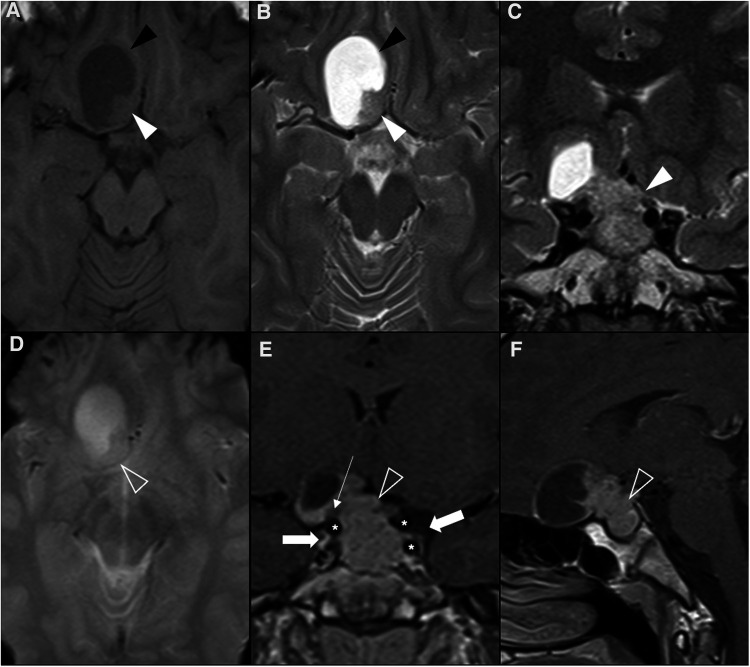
Prolactin-releasing pituitary neuroendocrine tumor in a 16-year-old boy presenting with headaches. Brain MRI including axial (A) T1WI as well as axial (B) and coronal (C) T2WI shows an expansile sellar and suprasellar lesion resembling a “snowman” appearance that cannot be differentiated from the pituitary gland, mainly isointense in T1WI and iso-hyperintense on T2WI (white arrowhead), and with a round hypointense T1 and hyperintense T2 cystic component (black arrowhead) located in the right anterior region and causing displacement of the right rectus and orbital gyri without associated edema. No associated paramagnetic foci suggestive of calcifications are depicted on axial (E) T2 gradient-echo imaging. Coronal (F) and sagittal (G) T1WI after gadolinium injection reveal homogenous enhancement of the solid component of the lesion (empty white arrowhead). There are no signs of cavernous sinus invasion, more prominent on the right (white arrows), without stenosis of the cavernous segments of the internal carotid arteries (asterisks). Note that the pre-chiasmatic segment of the right optic nerve is encased by the lesion [long white arrow in (F)], with significant adherence confirmed intraoperatively. Carotid arteries are marked with asterisks in (F).
